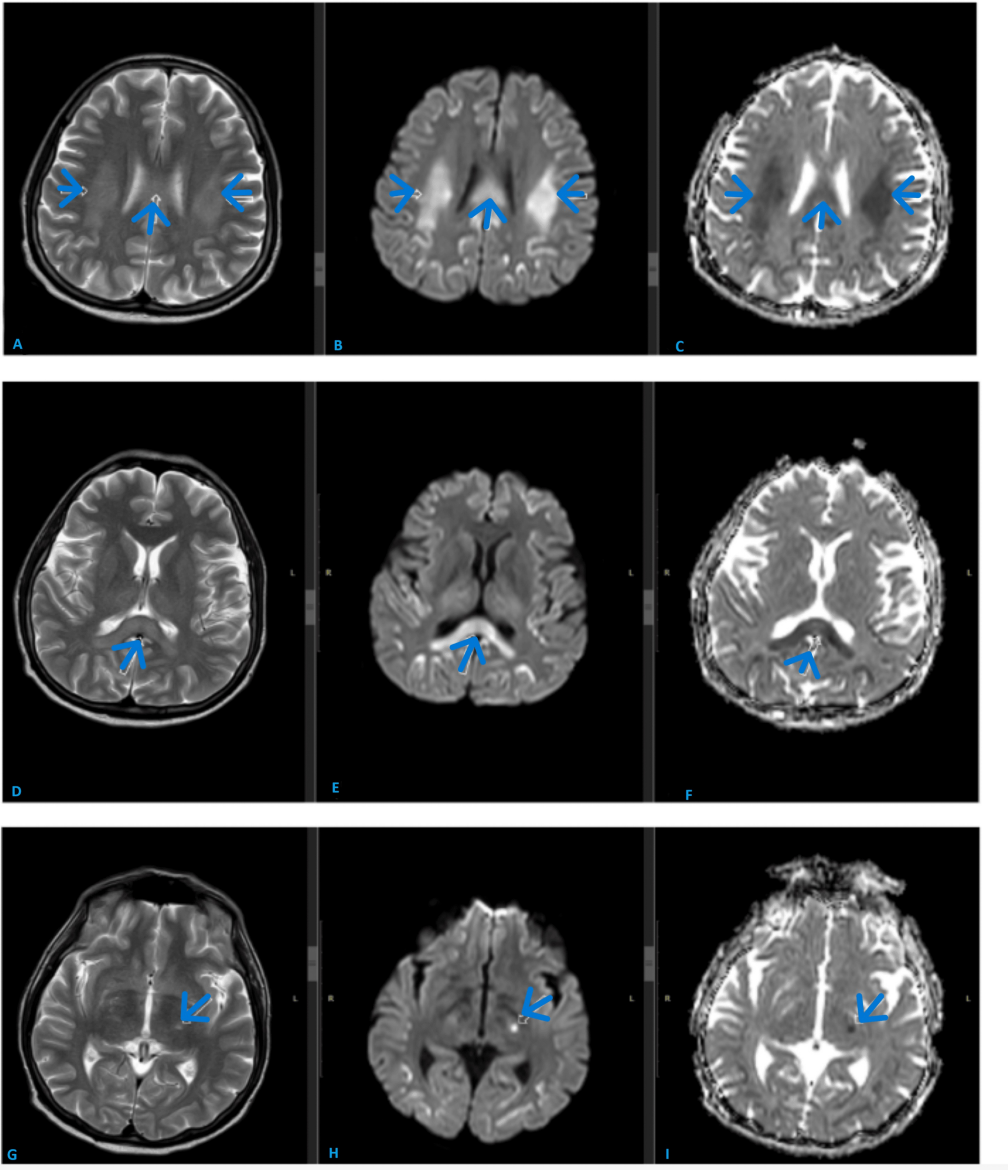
MR brain shows extensive abnormal T2 high signal with corresponding diffusion restriction in the bilateral frontoparietal white matter; trunk and splenium of corpus callosum and also a tiny focus in the left thalamus. Extensive abnormal T2 high signal is seen in the bilateral frontoparietal white matter(A);  trunk and splenium of corpus callosum (D) and also a tiny focus in the left thalamus (G). Corresponding diffusion restriction is also seen. Diffusion restriction is manifested as high signal on DWI sequences (B, E, and H) with low signal on ADC sequences (C, F, and I).
